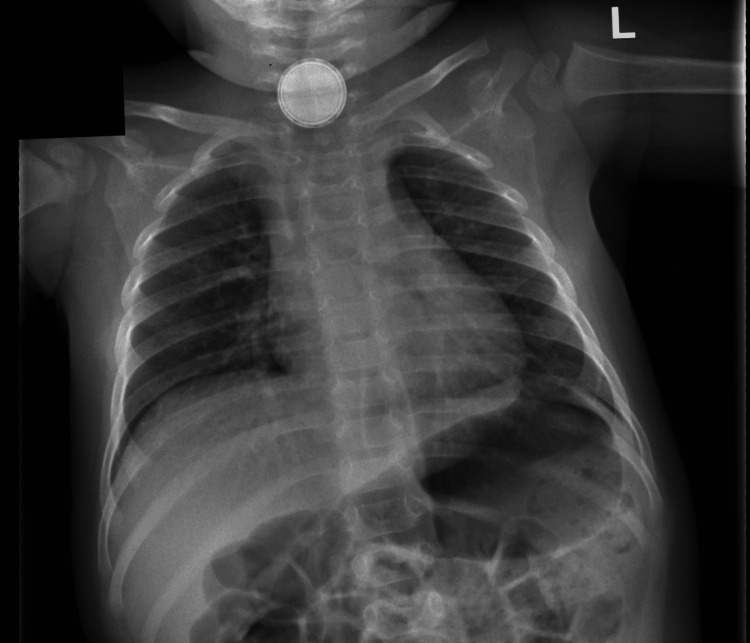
Anteroposterior (AP) erect chest X-ray
